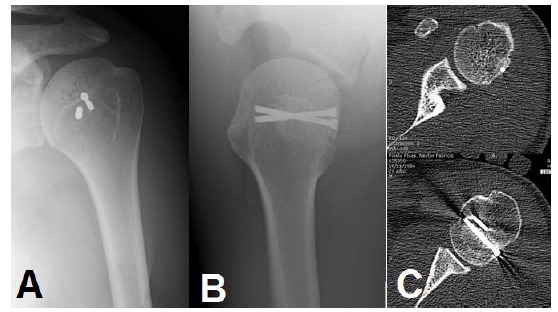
Humeral head augmentation: An osteochondral allograft is placed in the humeral defect and fixed with 2 headless compression screws (A,B). The CT scan shows adequate integration of the defect and restoration of the concavity (C).
